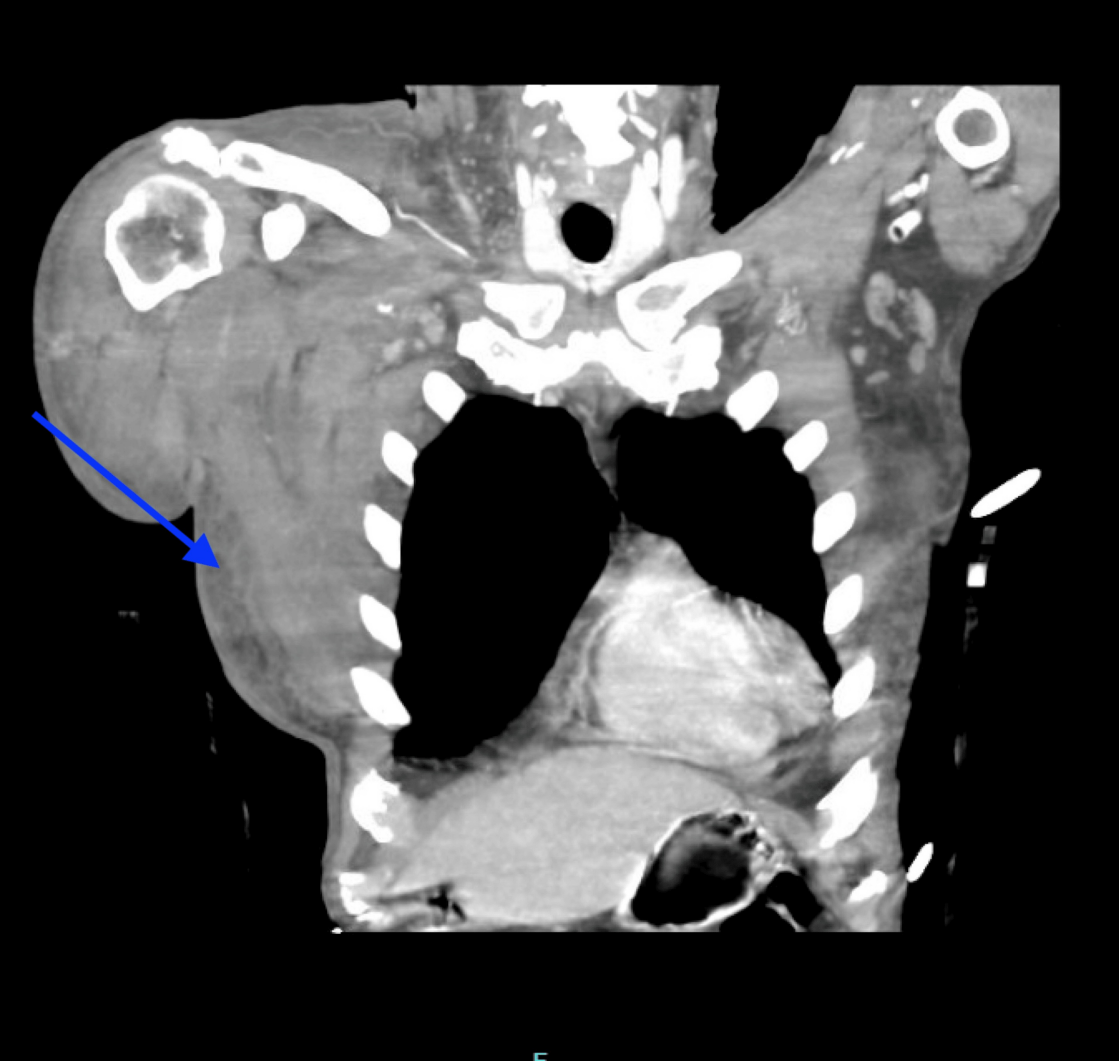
Computed tomography of the chest demonstrating a right chest wall hematoma (blue arrow).
